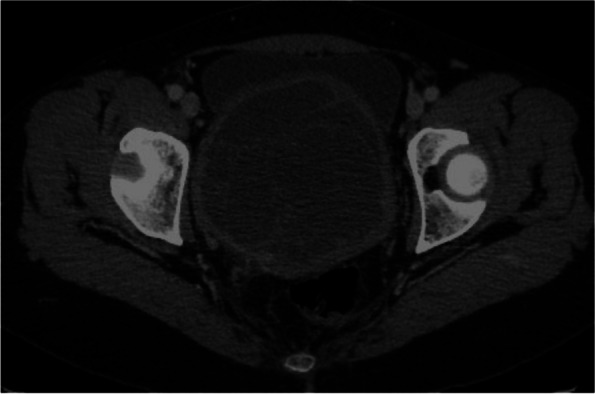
Axial CT image (IV contrast, portal phase). A large mass occupying the central part of the pelvis. It is a predominantly hypoattenuating mass with many thin septations and a thick well-delineated “capsule”. No invasion of the bladder or rectal wall was detected. No enlarged lymph nodes were present
